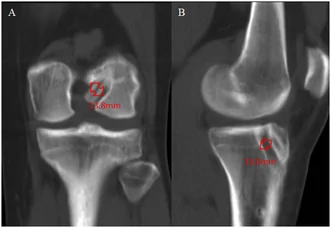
Preoperative CT assessment of femoral and tibial tunnel enlargement. Preoperative CT scans of the right knee in a supine patient. (A) Sagittal CT image showing femoral tunnel enlargement (arrow indicates tunnel), with a diameter of approximately 13.8 mm prior to revision. (B) Coronal CT image showing tibial tunnel widening (arrow indicates tunnel), measuring 14.0 mm. These findings guide surgical planning for autologous bone grafting. Orientation: sagittal and coronal planes; lateral view indicated with arrowhead. (CT, computed tomography.)
